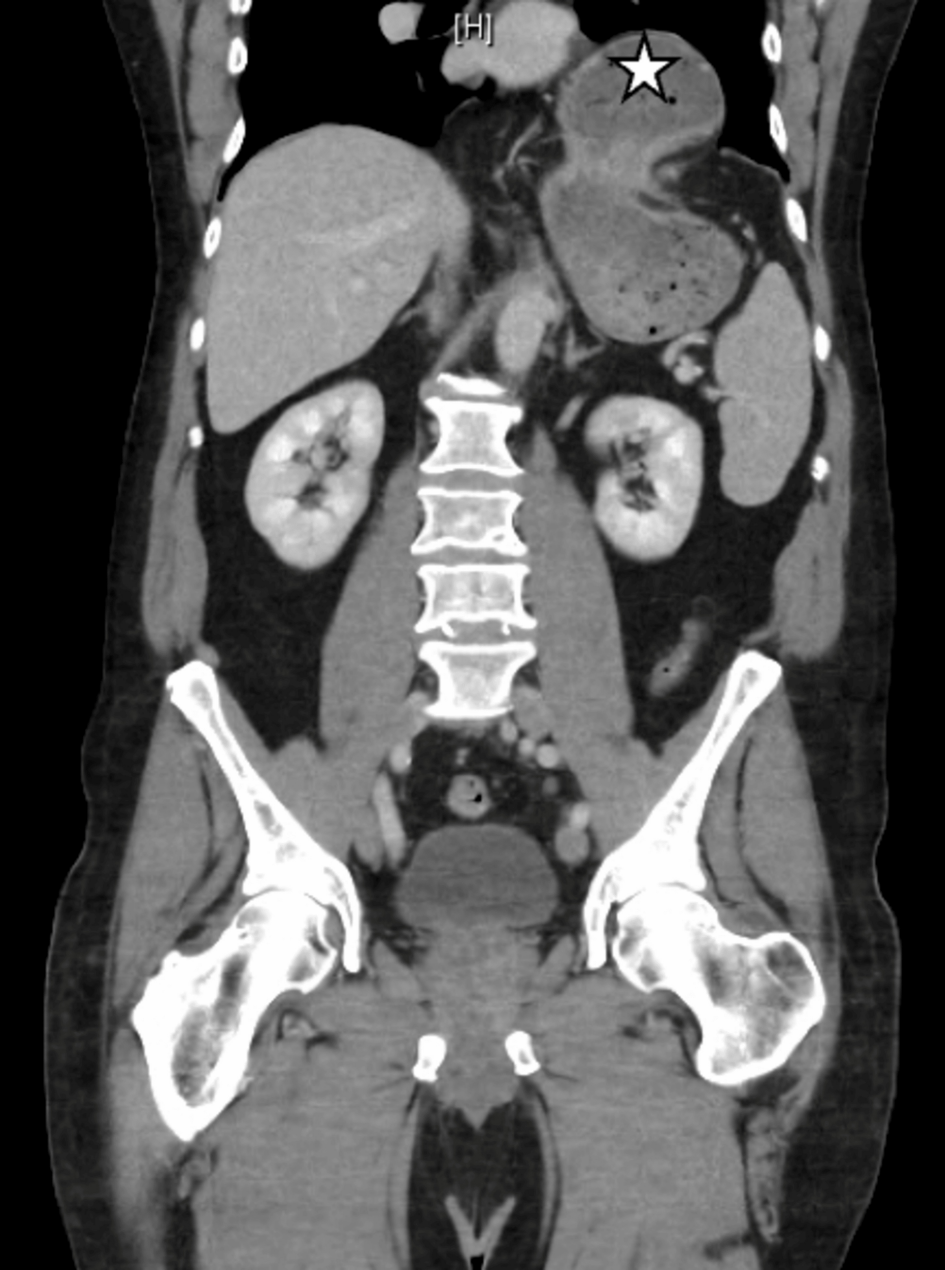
CT scan image of the abdomen The image demonstrates a large hiatal hernia (marked with a star).
